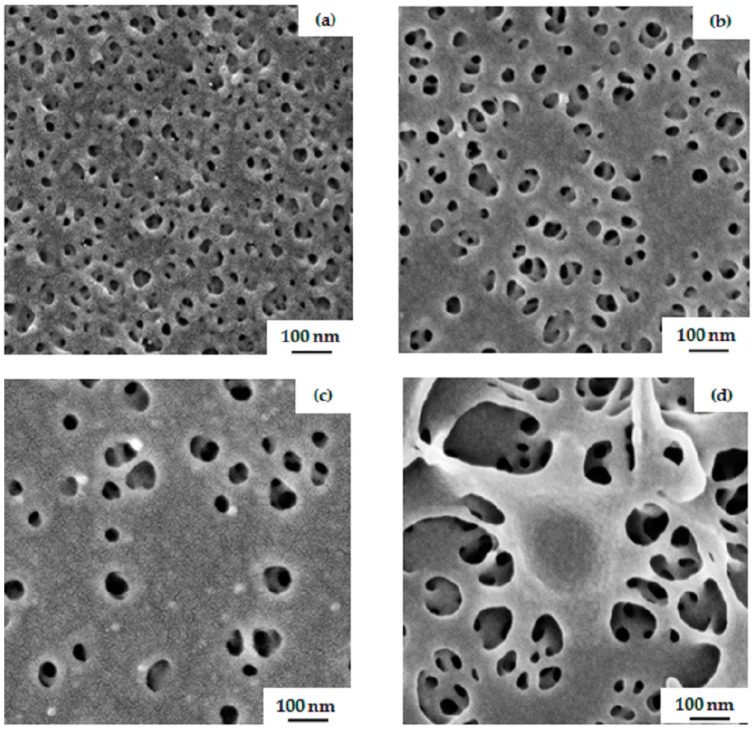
SEM images of surfaces of the membranes made from 24 wt% PS_81_-PSMA_19_^170^ in THF/DMF 50/50 wt %. Evaporation time before immersion: (a) 5 s, (b) 10 s, (c) 20 s, (d) 25 s.
